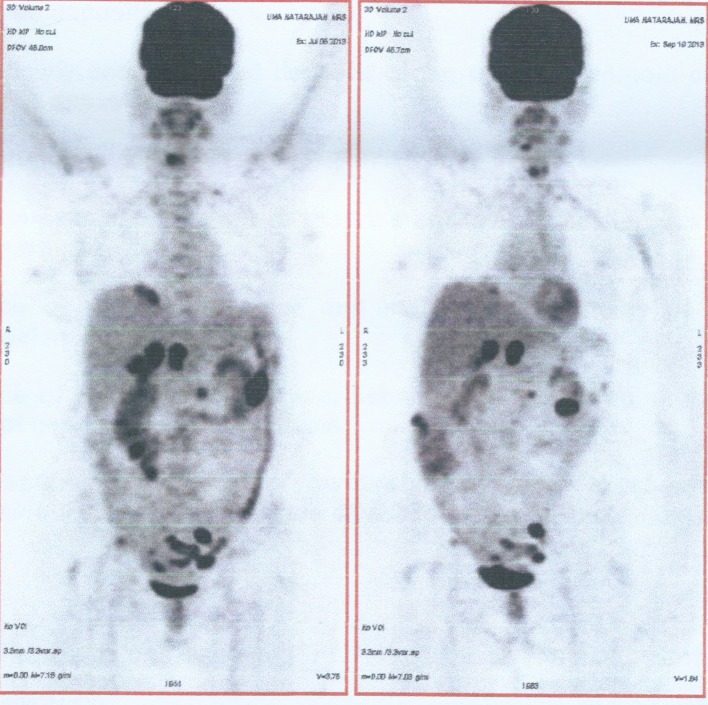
Comparision of whole body FDG PET CT scan before and after chemotherapy.
